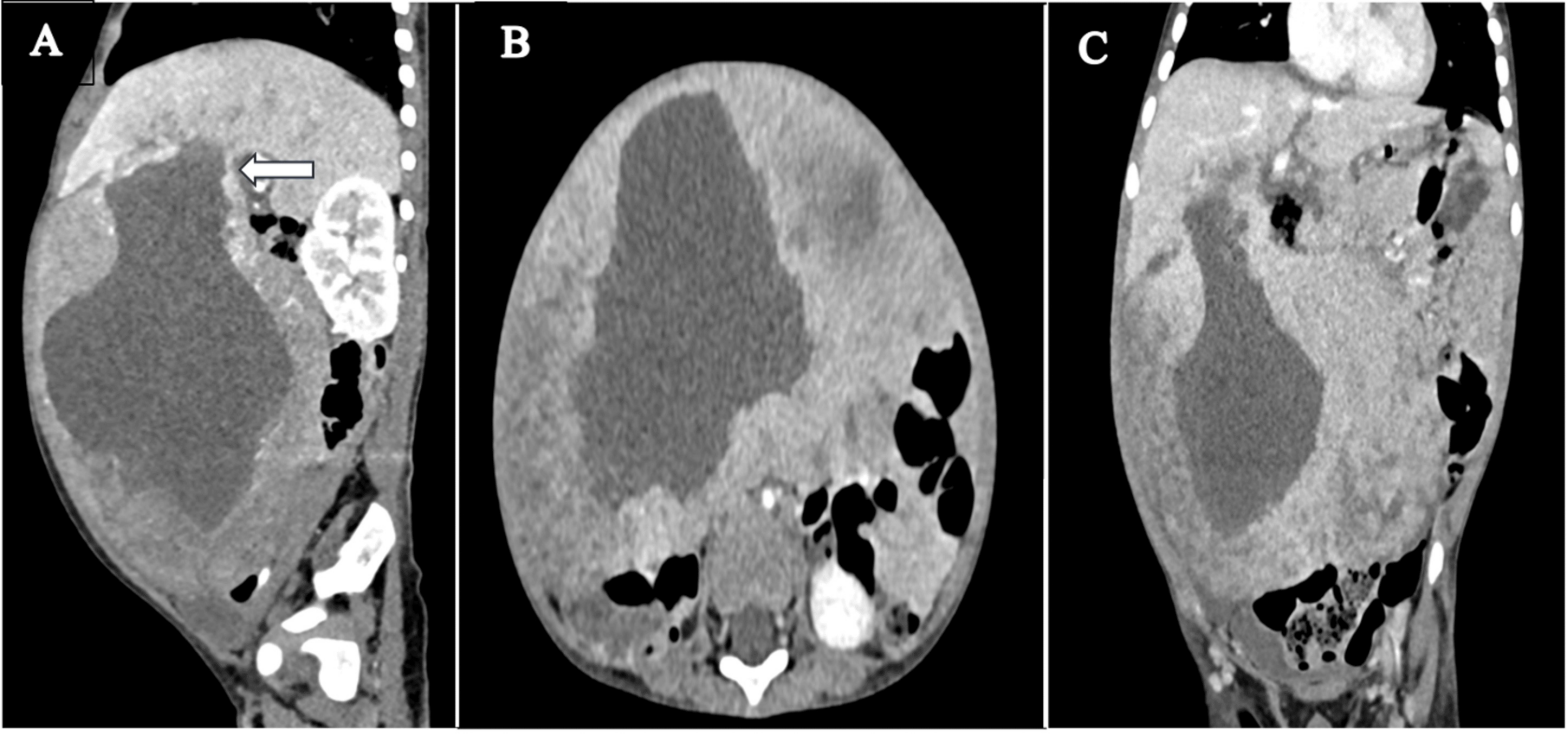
Contrast-enhanced computed tomography images depicting a large heterogenous mixed solid-cystic mass (cystic predominant) arising from the liver and protruding into the abdominal cavity. (A) Sagittal image showing exophytic solid cystic mass arising from the inferior aspect of the right lobe of the liver (white arrow). (B) Axial image showing mass causing mass effect with the displacement of the bowel loops and abutting anterior abdominal wall muscles. (C) Coronal image showing mass with enhancing solid component and interspersed non-enhancing cystic component.
